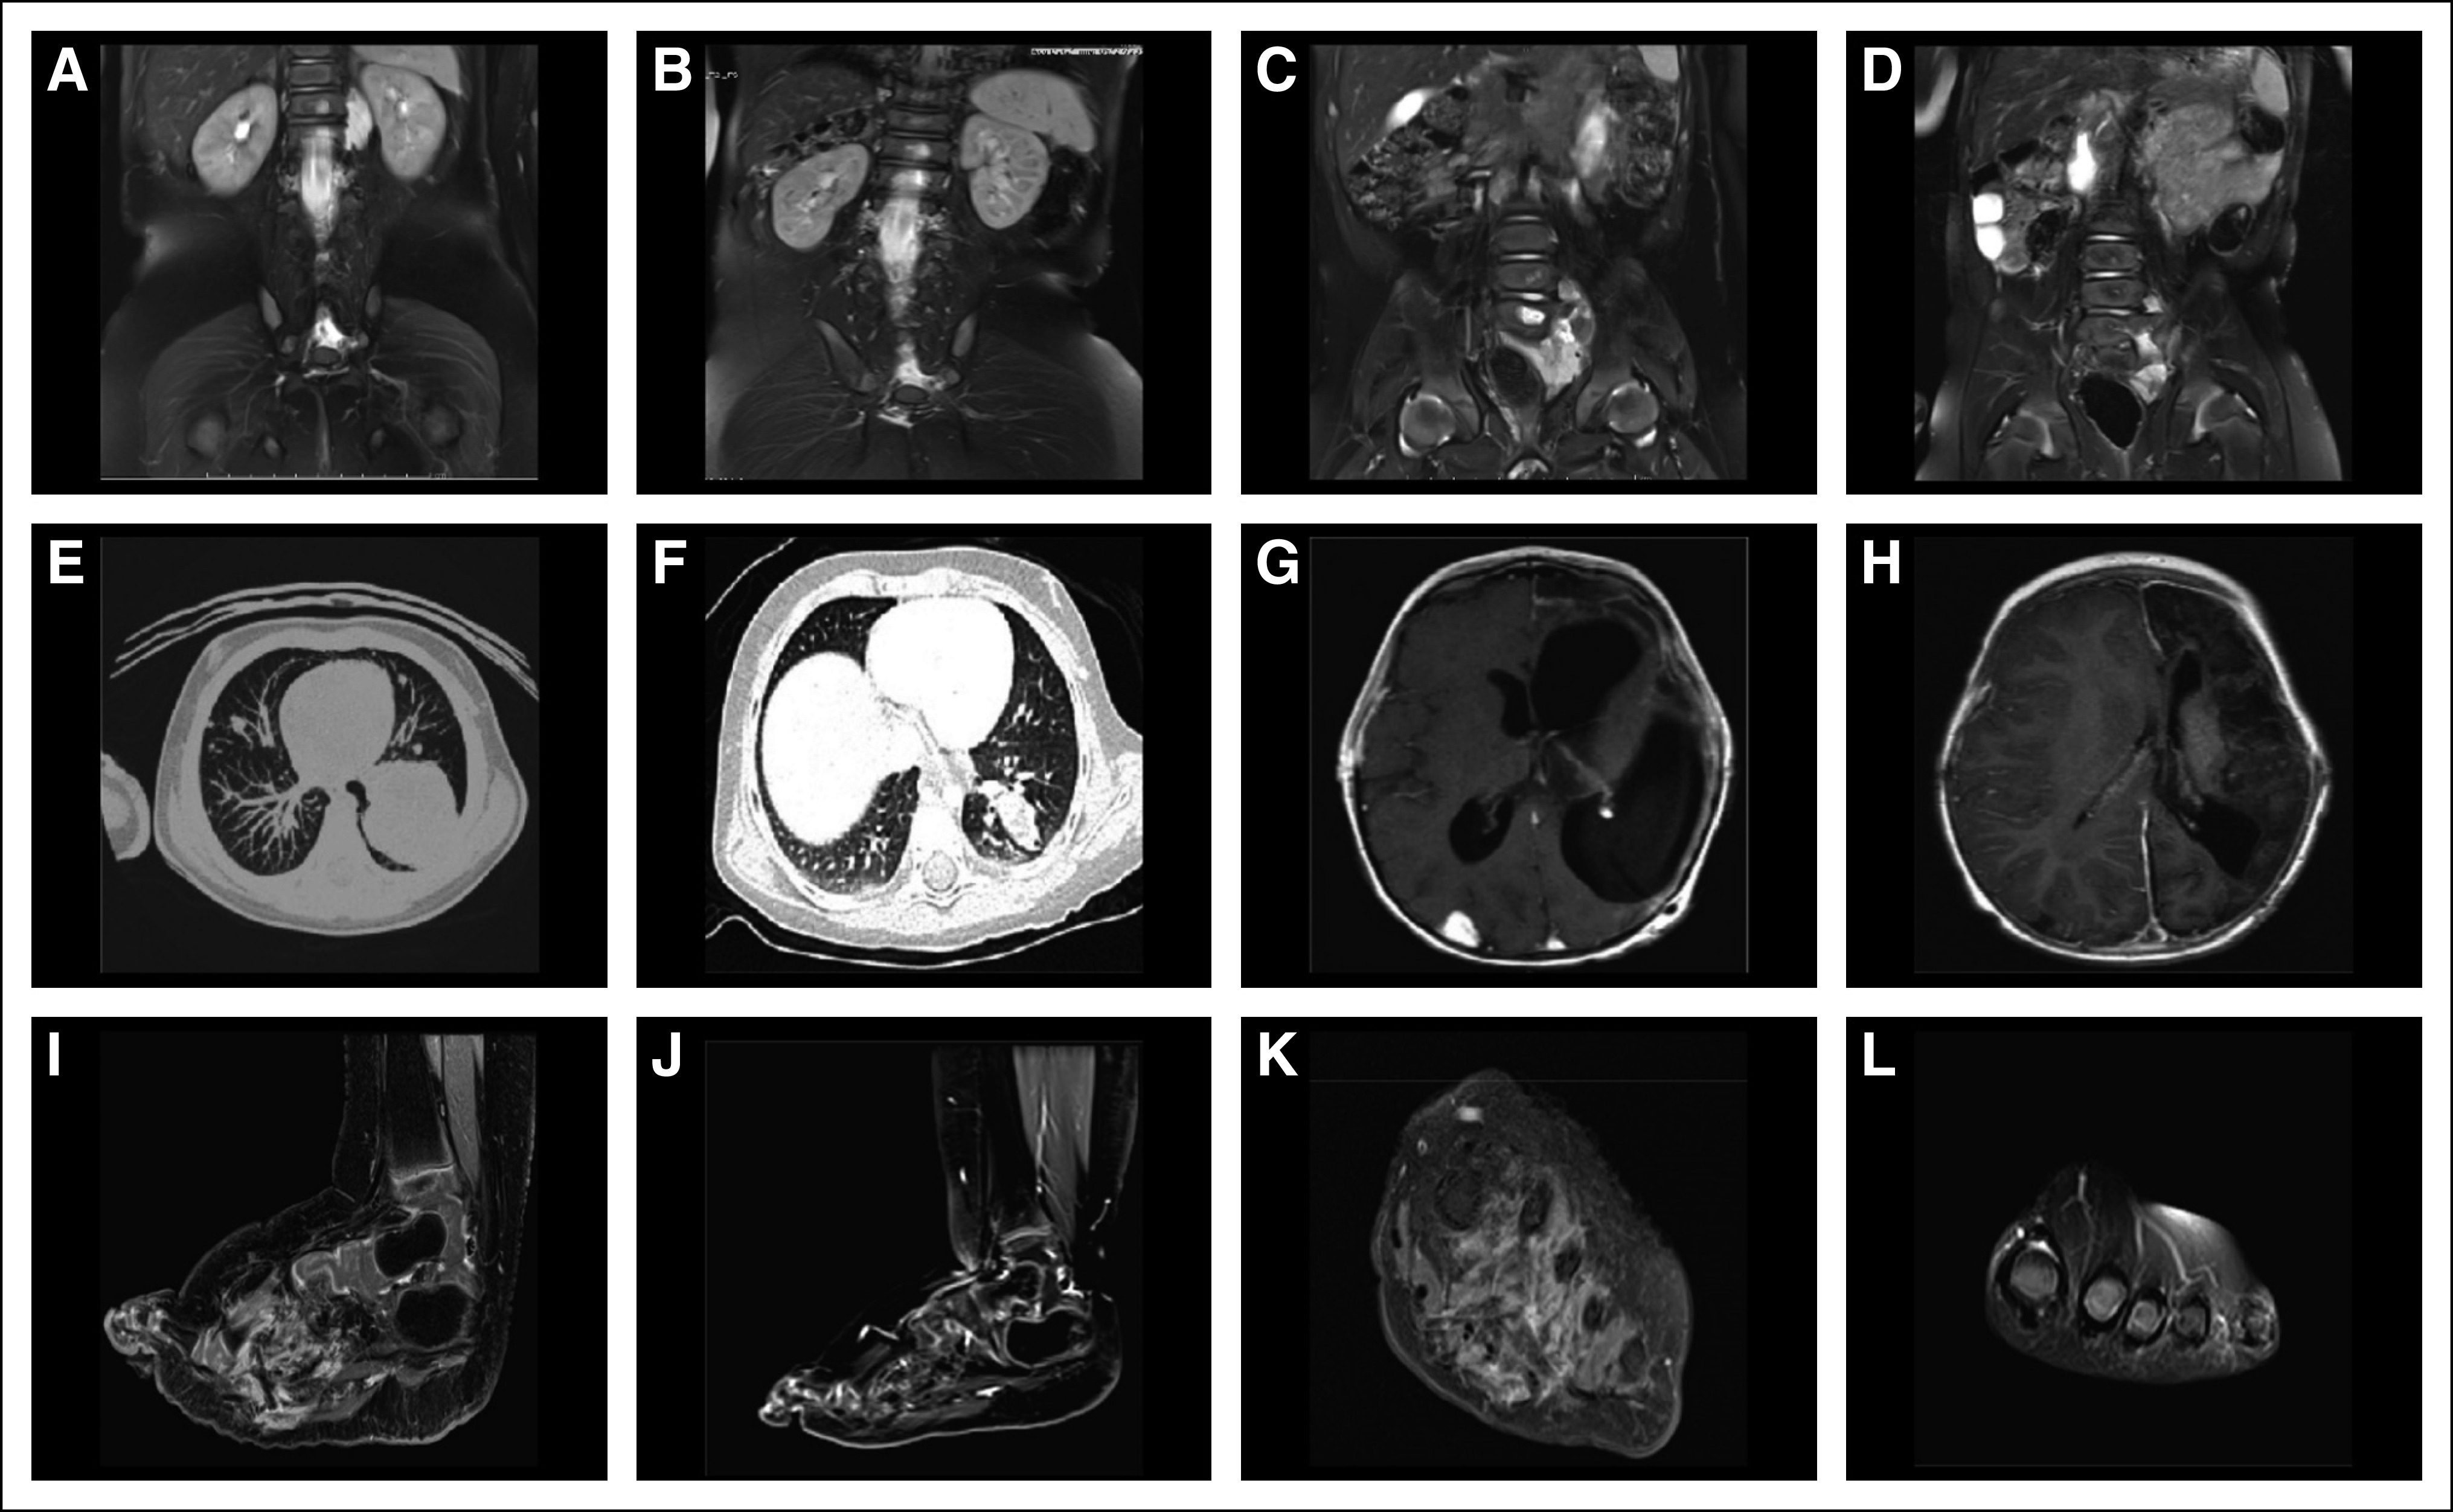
Selpercatinib activity in patients with soft-tissue sarcoma. Patient 3: (A, C) computed tomography (CT) scans of the abdomen at baseline and (B, D) after 6 months of treatment with selpercatinib, revealing multiple paraspinal retroperitoneal and pelvic lesions in a patient with infantile myofibroma/hemangiopericytoma harboring an *MYH10-RET* fusion. A partial response was observed after one cycle of selpercatinib; after six cycles, the paraspinal lesion had completely resolved, and the patient regained lower extremity neurologic function. Patient 4: CT scans at baseline of (E) the lungs and (G) brain and after 8 months of treatment with selpercatinib of (F) the lungs and (H) brain in a patient with an *SPECC1L-RET* fusion–positive congenital mesoblastic nephroma and infantile fibrosarcoma. After two cycles of selpercatinib, a partial response was observed with a 41% tumor reduction, which deepened to 66% by cycle 8. Patient 5: CT scans (I, K) at baseline and (J, L) after 2 months of treatment with selpercatinib of the left foot in a patient with an *NCOA4-RET* fusion–positive lipofibromatosis. Selpercatinib treatment resulted in a significant decrease in tumor burden leading to improvements in gait and locomotion.
